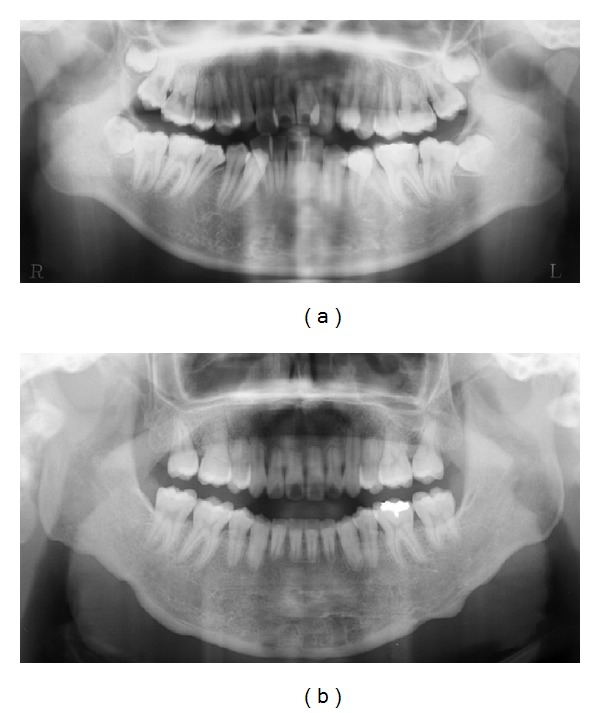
Orthopantomograph. (a) Pretreatment. (b) Posttreatment. Slight resorption of the roots of the lower incisors is observed but does not appear clinically significant.
